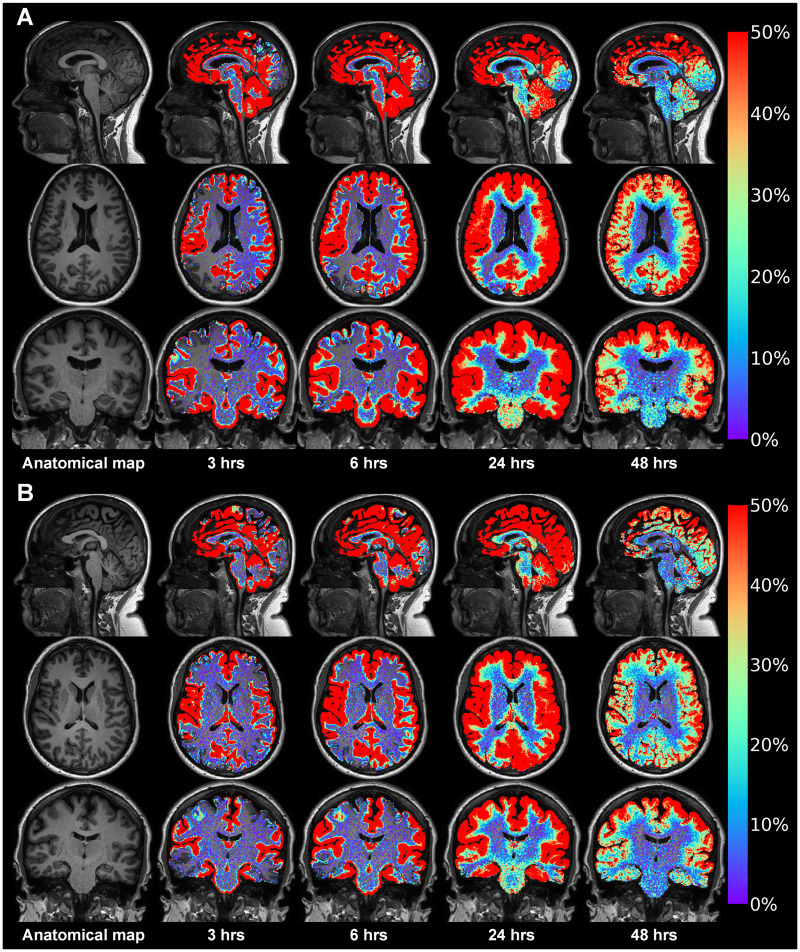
Time course of parenchymal distribution of CSF tracer in an IIH patient and a REF subject. gMRI from an IIH patient who complained of headache, visual obscurations, blurred vision, pulsatile tinnitus, olfactory dysfunction, dizziness and subjective cognitive impairment in A. The pulsatile ICP was pathological (MWA 6.6 mmHg on average), while static ICP was within normal range (average of mean ICP 12.1 mmHg). A REF patient at comparable age who complained of headache, dizziness and cognitive impairment in B. There was no sign of papilledema, and overnight ICP was normal. In both these patients, the CSF tracer (gadobutrol) was injected intrathecally and MRI obtained after 3 h, 6 h, 24 h and 48 h. The images are post-processed using FreeSurfer software, and showing percentage increase in normalized T1 signal over time (see colour scale to the right). Tracer in CSF spaces has been subtracted, thus only presenting tracer enrichment within brain tissue. After 3 h and 6 h, tracer enriches most strongly in brain regions adjacent to large artery trunks at the brain surface, including the medial temporal lobe close to the posterior cerebral arteries, the cingulum adjacent to the anterior cerebral arteries in the anterior interhemispheric fissure and the Sylvian fissure, where the middle cerebral artery trunks reside. Notably, the tracer enriches the extra-vascular space of the brain from outside and inward (centripetally), with peak enhancement at about 24 h.
